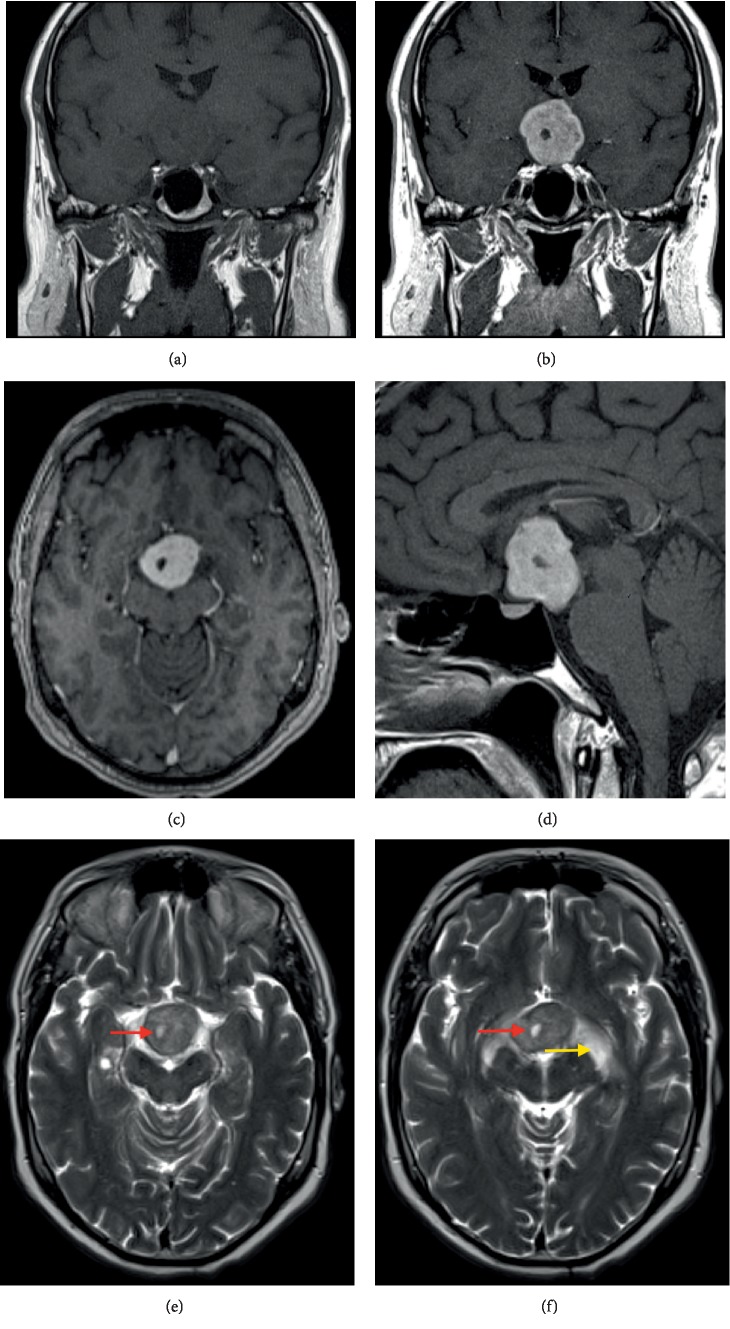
Brain MRI (a) large suprasellar mass T1-isointense to grey matter. (b–d) Vivid homogeneous T1 contrast enhancement of the lesion. (e) Axial T2-weighted images show a iso-to-hyperintense mass with a small cystic component (red arrows). (f) The mass compresses the optic chiasm, with oedematous swelling of the chiasm and both optic tracts (yellow arrow).
